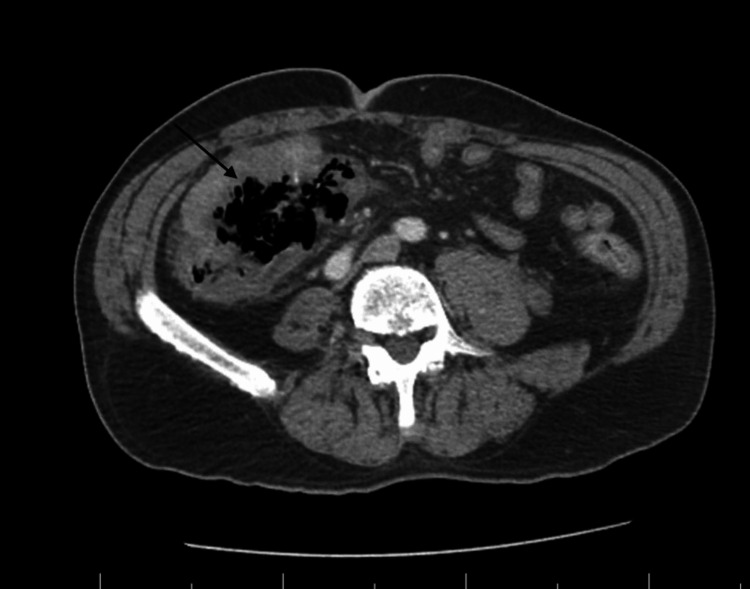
CT Abdomen/Pelvis: Arrow Points to Colon Perforation
